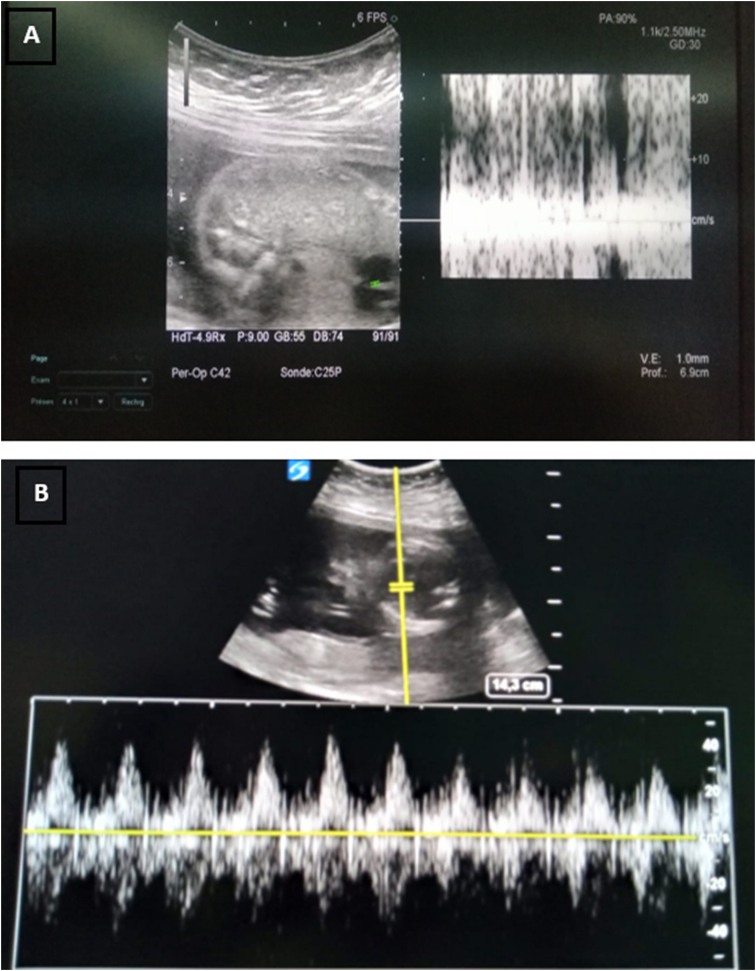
Obstetrical ultrasound showing the presence of fetal cardiac activity postoperatively (immediate: image A, and after 6 hours image B).
